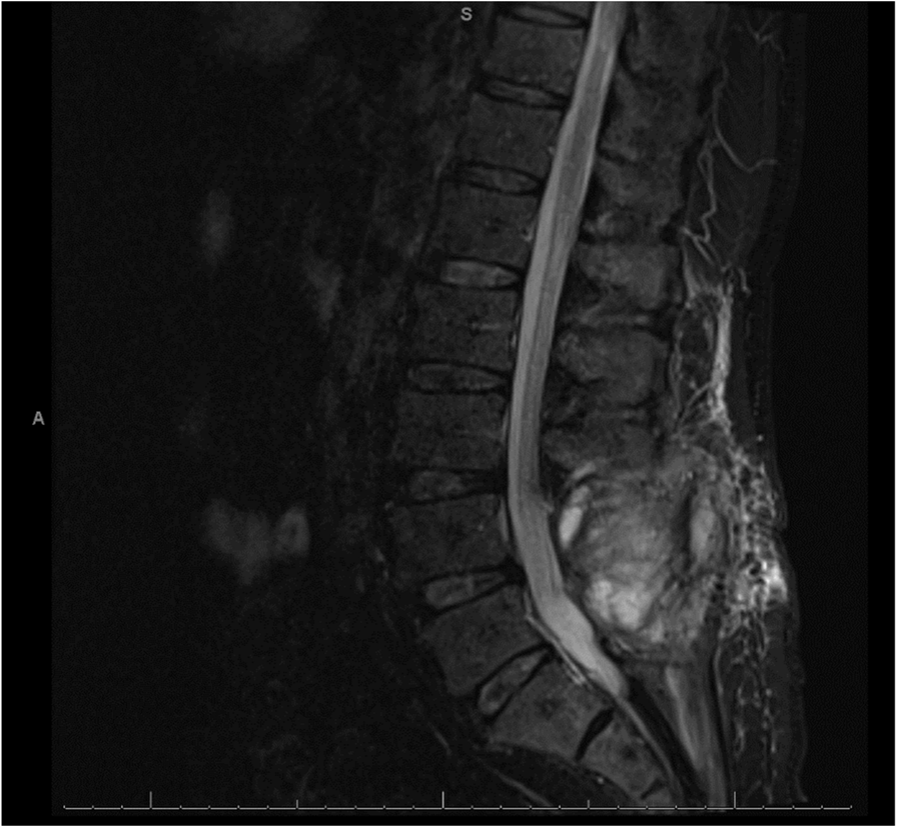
Non-contrast 1.5 T MRI STIR sequence of the lumbar spine, taken after surgery and prior to the blood patch procedure
